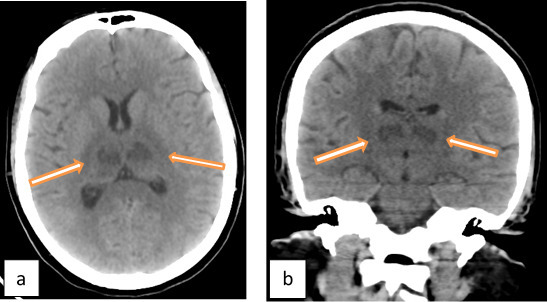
CT brain was done on day 4. (a) Axial image at the level of thalami and (b) coronal image at the level of thalami showing abnormal hypodensity with swelling at both thalami (arrows).
